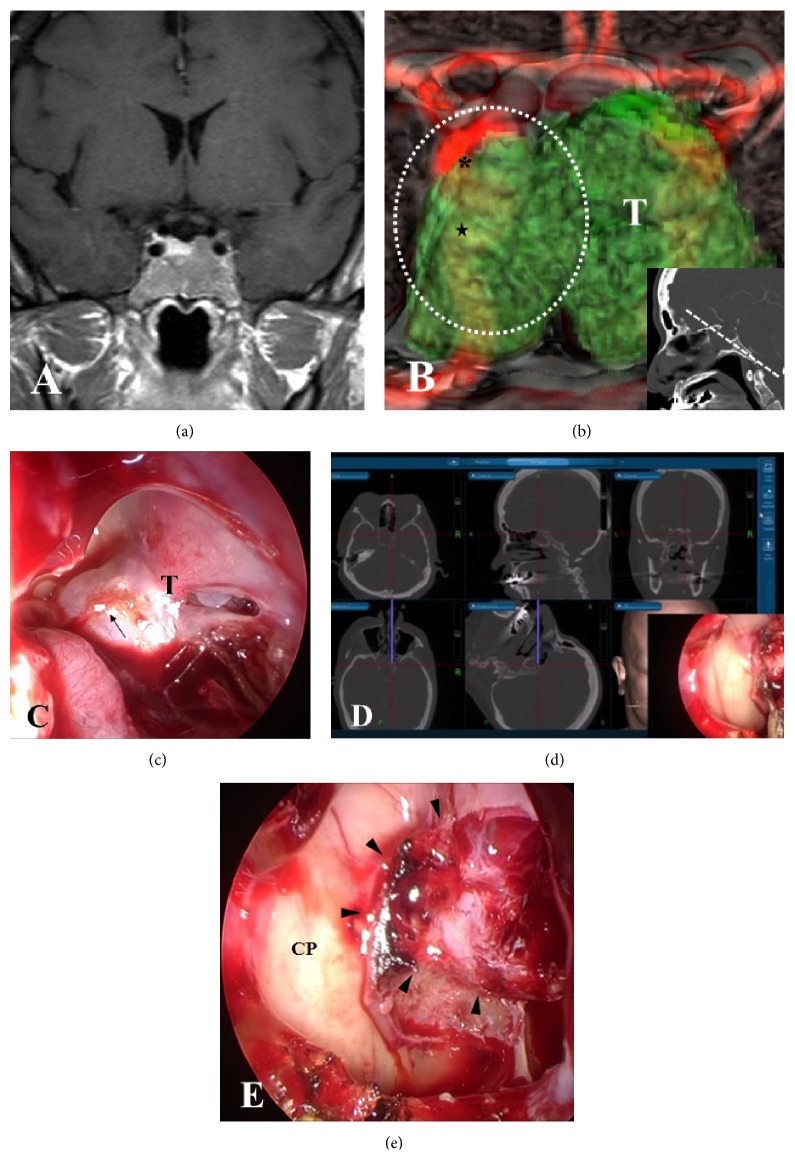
Preoperative image of a fusion model of 3D-CT and MRI and intraoperative endoscopic view in Case 1. (a) Preoperative coronal Gd-enhanced MRI in Case 1 showing a pituitary tumor with extensive invasion into the sphenoidal sinus. (b) Preoperative 3D-CT and MRI fusion model demonstrating that bilateral ICAs (C3 and C4 portions) are buried in the invasive tumor at the anterior plane of the sella (inset) (stars: C4; asterisks: C3). (c) Endoscopic view before tumor resection showing the massive tumor that widely protruded into the sphenoidal sinus. (d) A neuronavigation system demonstrating the locations of the ICAs and the destroyed sella in the endoscopic view (c). CT images on the navigation display show the position of the chip and trajectory of the flexible probe that was placed on the marked position (black arrow) on the endoscopic view (c). (e) Endoscopic view after resection of the tumor showing the widely eroded sellar floor occupied with residual intrasellar tumor and intact bony structures over the ICAs (arrowheads: eroded sellar floor; CP: ICA prominence). T: tumor; the area of white-dashed line: endoscopic view in (c).
